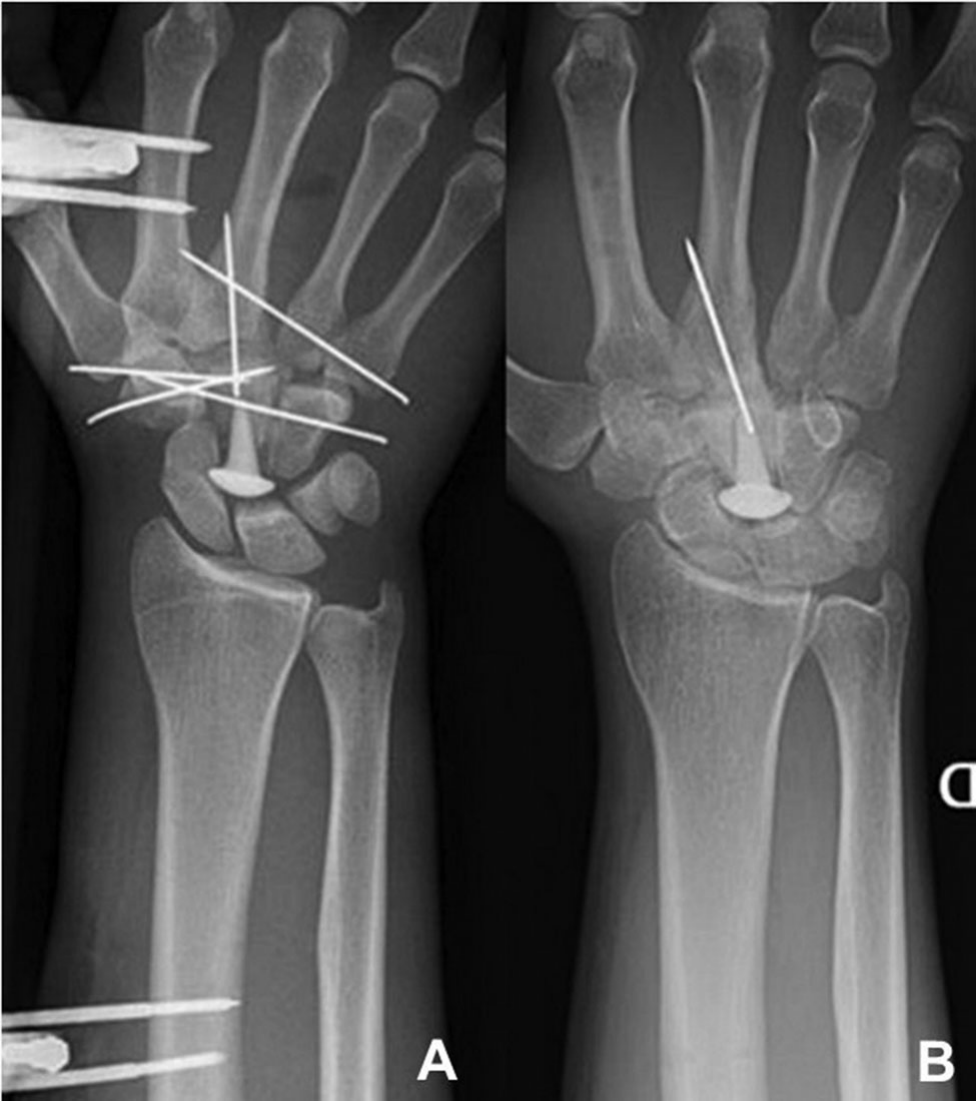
(A) Post-operative x-ray case 2. (B) X-ray at 29 months’ follow-up, after removal of the external fixation and K-wires.
